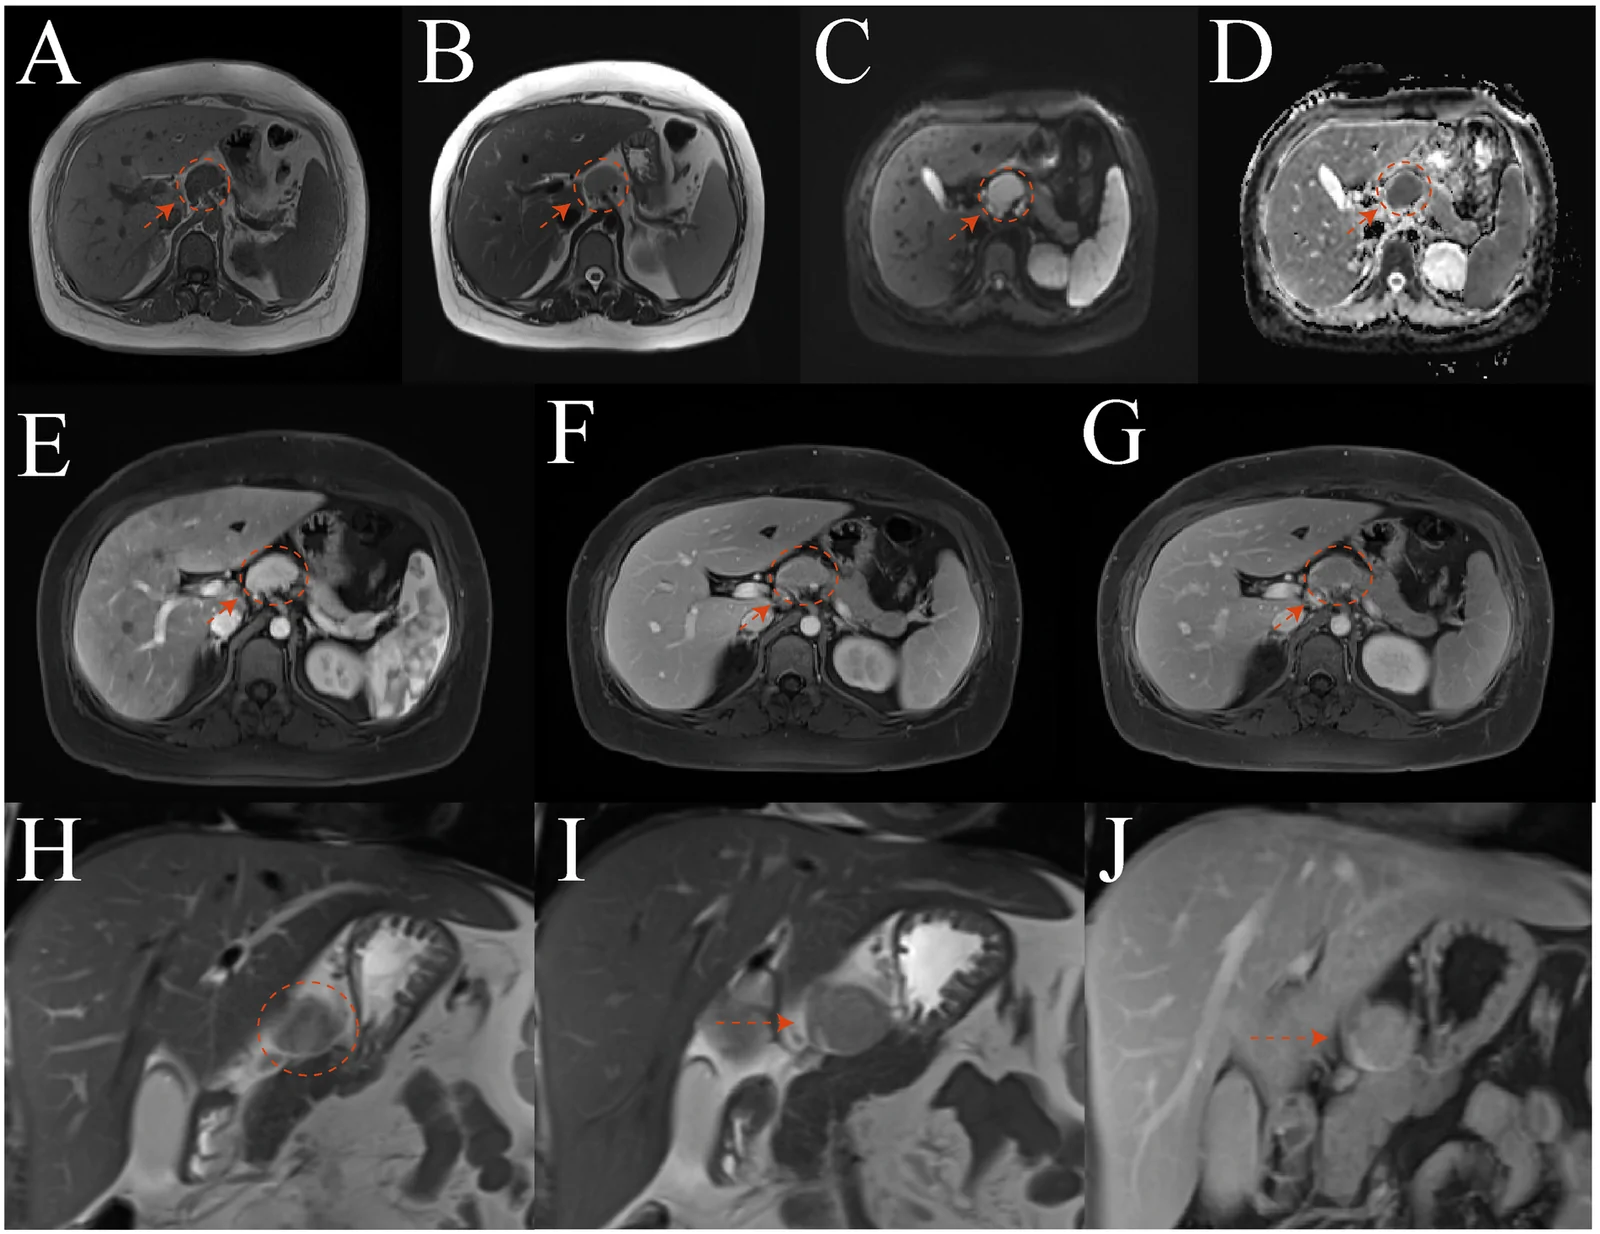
Magnetic resonance imaging findings of the peripancreatic mass. The lesion was hypointense on axial T1-weighted imaging (A) and mildly hyperintense on axial T2-weighted imaging (B) Diffusion-weighted imaging showed hyperintensity (C), with corresponding hypointensity on the apparent diffusion coefficient map (D); the mean ADC value was approximately 0.73 × 10⁻^3^ mm^2^/s, consistent with restricted diffusion. Dynamic contrast-enhanced MRI demonstrated marked arterial-phase enhancement (E), followed by relatively lower enhancement during the portal venous (F) and delayed phases (G) Coronal T2-weighted images from adjacent sections (H,I) localized the lesion to the superior border of the pancreatic body. On retrospective review, a subtle intervening tissue plane between part of the lesion and the pancreatic surface was identified (arrow in I), although a complete radiological capsule could not be confidently identified. The delayed-phase contrast-enhanced coronal image (J) further demonstrated close apposition of the lesion to the pancreatic surface without convincing evidence of intrapancreatic origin. Red dashed circles and arrows identify the lesion; in panel I, the arrow specifically indicates the lesion–pancreas interface.
